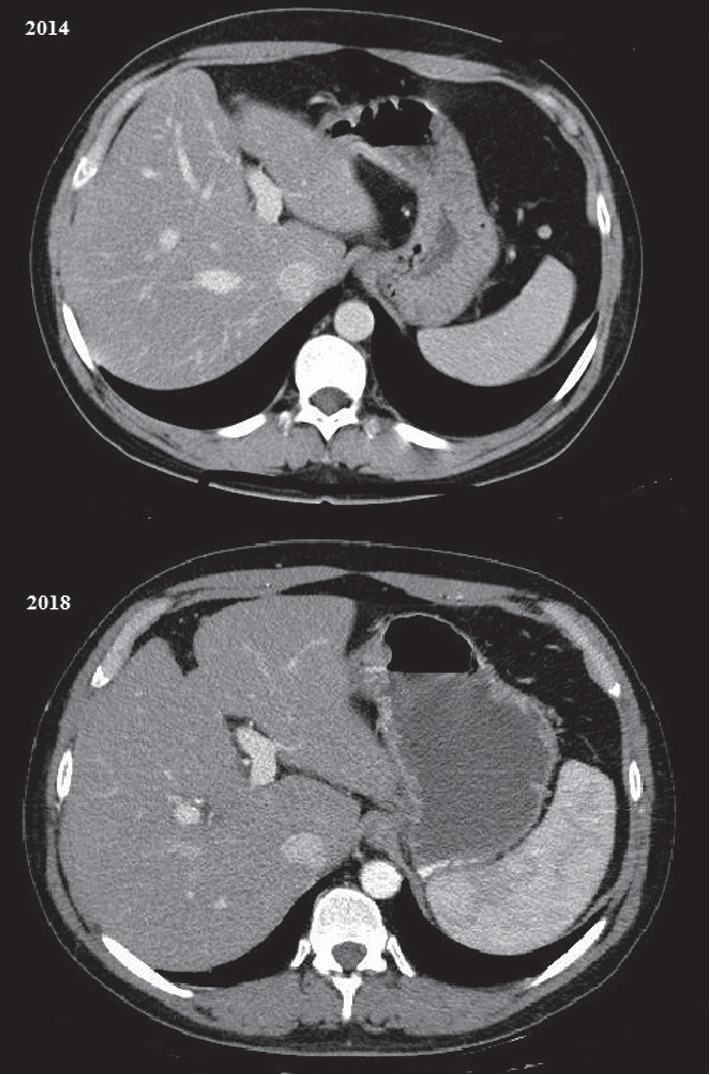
Comparison between arterial-phase abdomen CT scans from 2014 (before AZA therapy) and 2018 (after AZA therapy).
